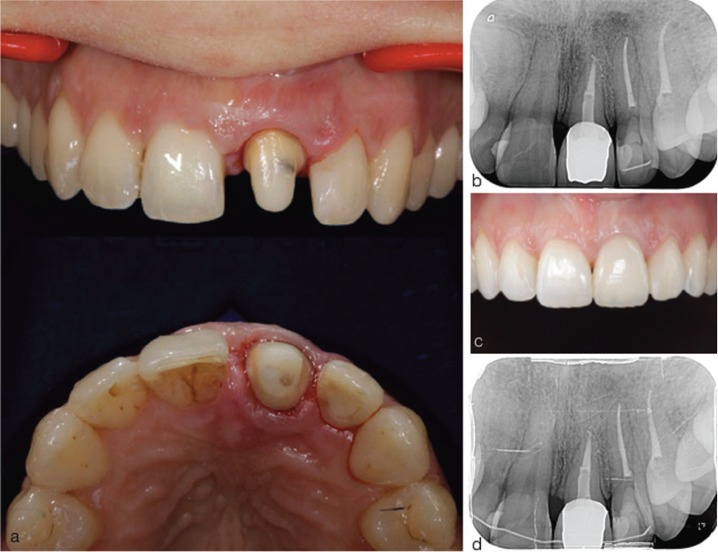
(a) Crown preparation (b) post-operative radiograph (c) post-operative labial view (d) radiograph taken after 4 years of treatment.
